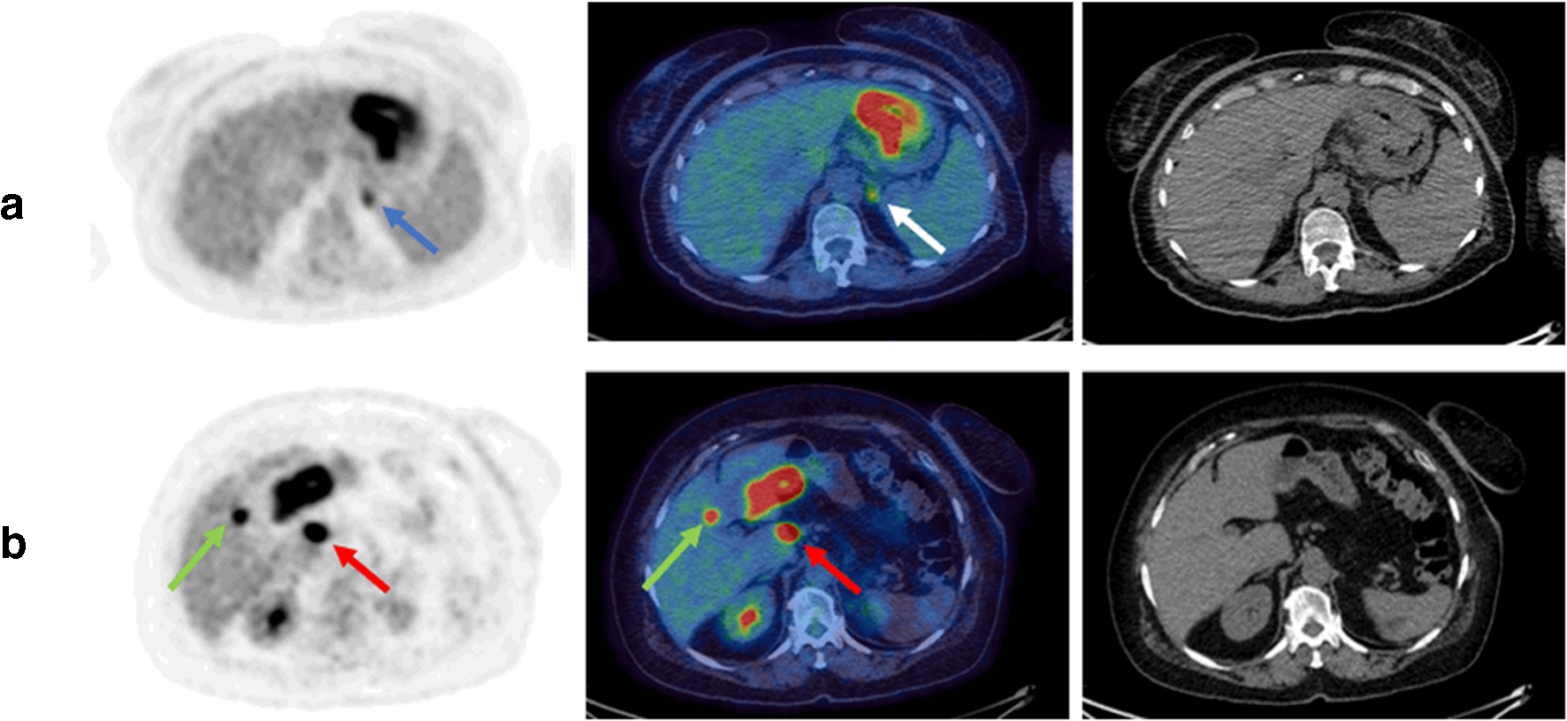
Example FDG PET-CT images from patients with occult metastases. Shown are axial images, from left to right: MIP, fused PET-CT, CT only; (a) positive gastric primary plus occult adrenal metastasis (*blue and white arrows*), (b) positive gastric primary with porta hepatis node (*red arrows*) plus occult hepatic metastasis (*green arrows*)
